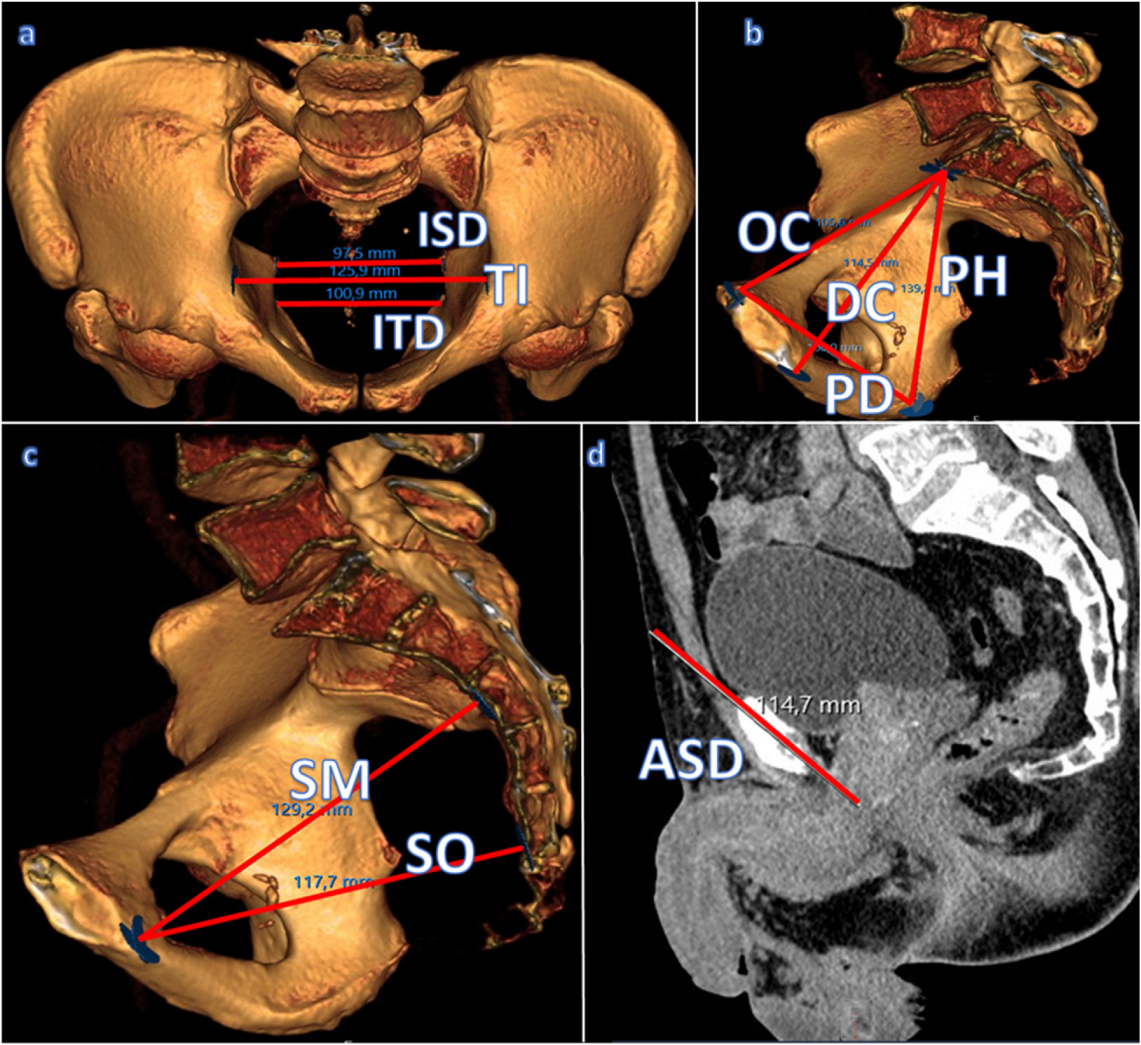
Pelvic parameters measured with CT scan; (a) interspinous distance, transverse inlet, and intertuberous distance; (b) obstetric conjugate, pelvic height, pelvic depth, and diagonal conjugate; (c) sagittal midpelvic and sagittal outlet; (d) apex-skin distance.
